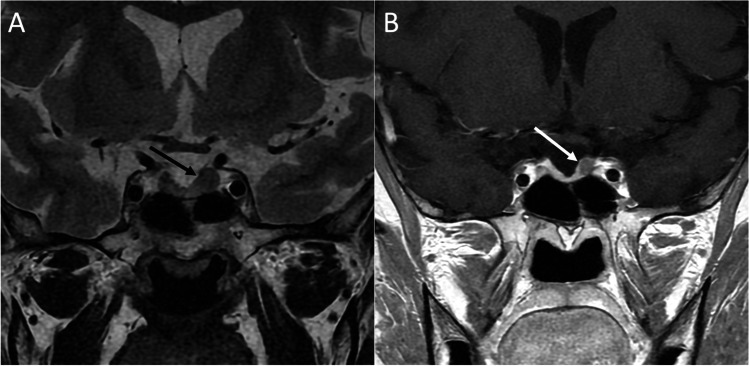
Growth hormone–secreting pituitary microadenoma. Coronal T2- (A) and contrast-enhanced T1-weighted (B) images show a mild thickening of the left aspect of the pituitary gland (black arrow) due to the presence of a small round lesion enhancing less than the remaining glandular parenchyma (white arrow)
